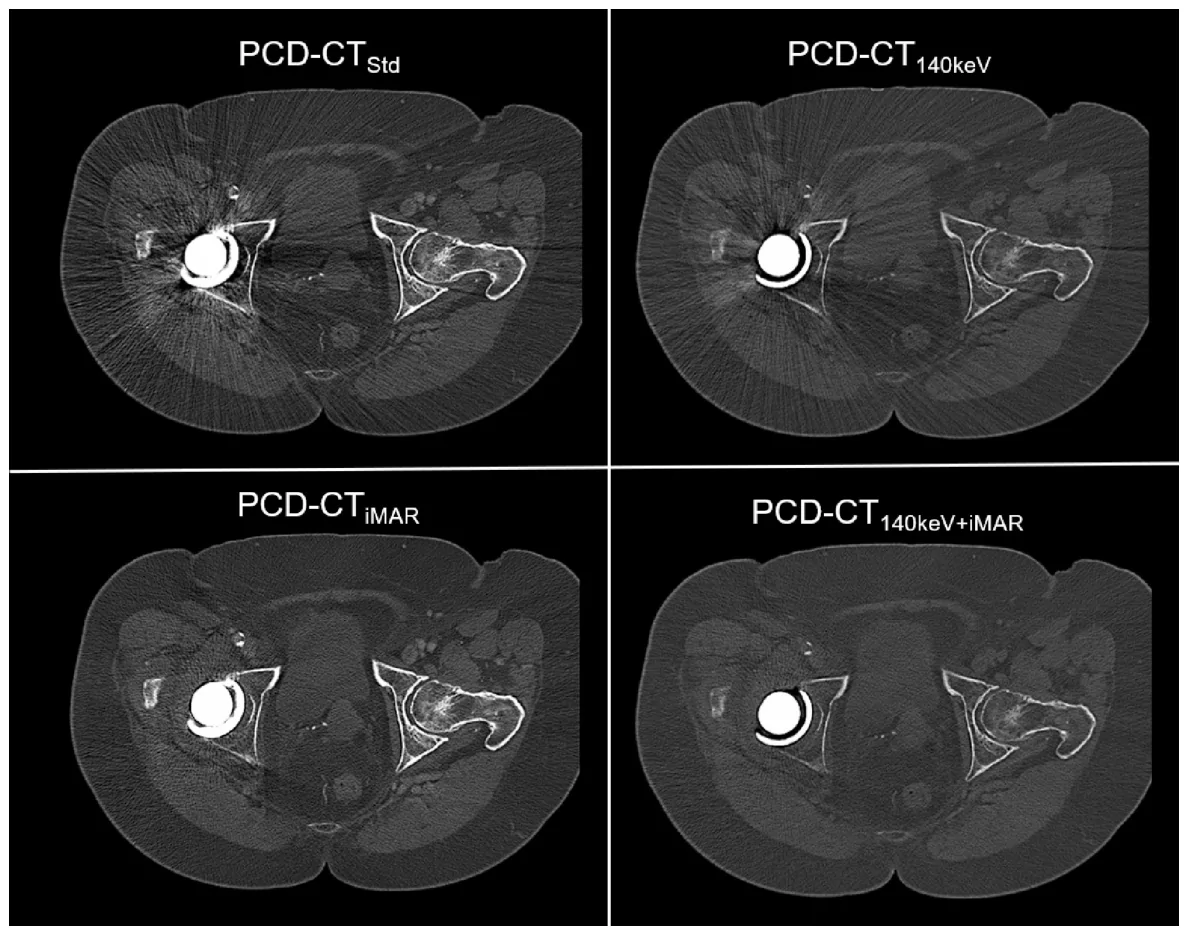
Example images from a 71-year-old patient with non-small cell lung cancer and a right hip prosthesis. PCD-CT at 140 keV achieved only limited metal artifact reduction, whereas iMAR alone and iMAR combined with 140-keV virtual monoenergetic reconstruction produced substantially greater artifact suppression. PCD-CTStd indicates standard reconstruction; PCD-CT140keV, virtual monoenergetic reconstruction at 140 keV; PCD-CTiMAR, dedicated iterative metal artifact reduction; and PCD-CT140keV+iMAR, the combination of iMAR with 140-keV virtual monoenergetic reconstruction [89].
